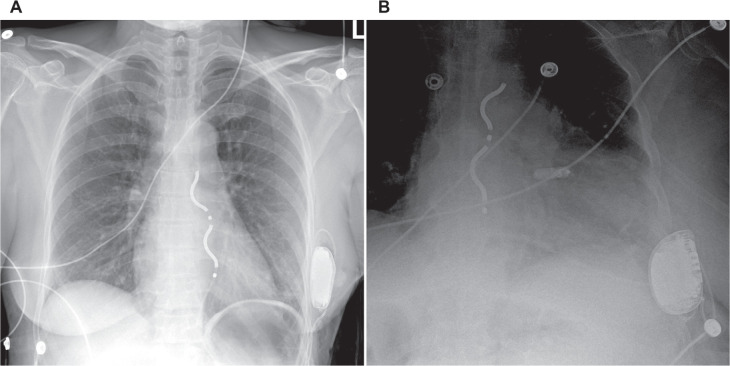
A: Frontal chest radiograph of an extravascular implantable cardioverter-defibrillator (EV-ICD) that includes a substernal lead. B: An EV-ICD with a separate leadless right ventricular pacemaker.
